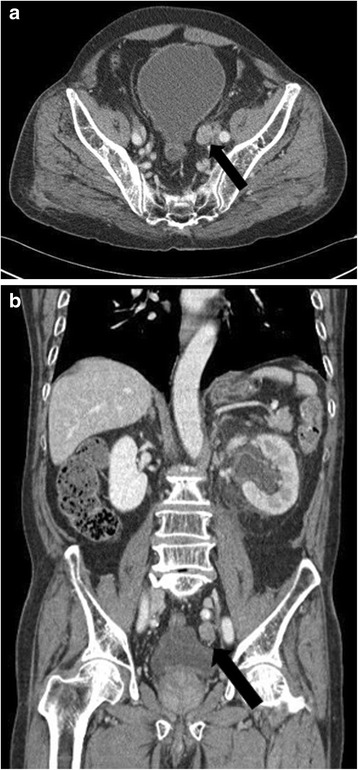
Abdominal computed tomography showing a left ureteral mass with hydronephrosis. a axial view, b coronal view
